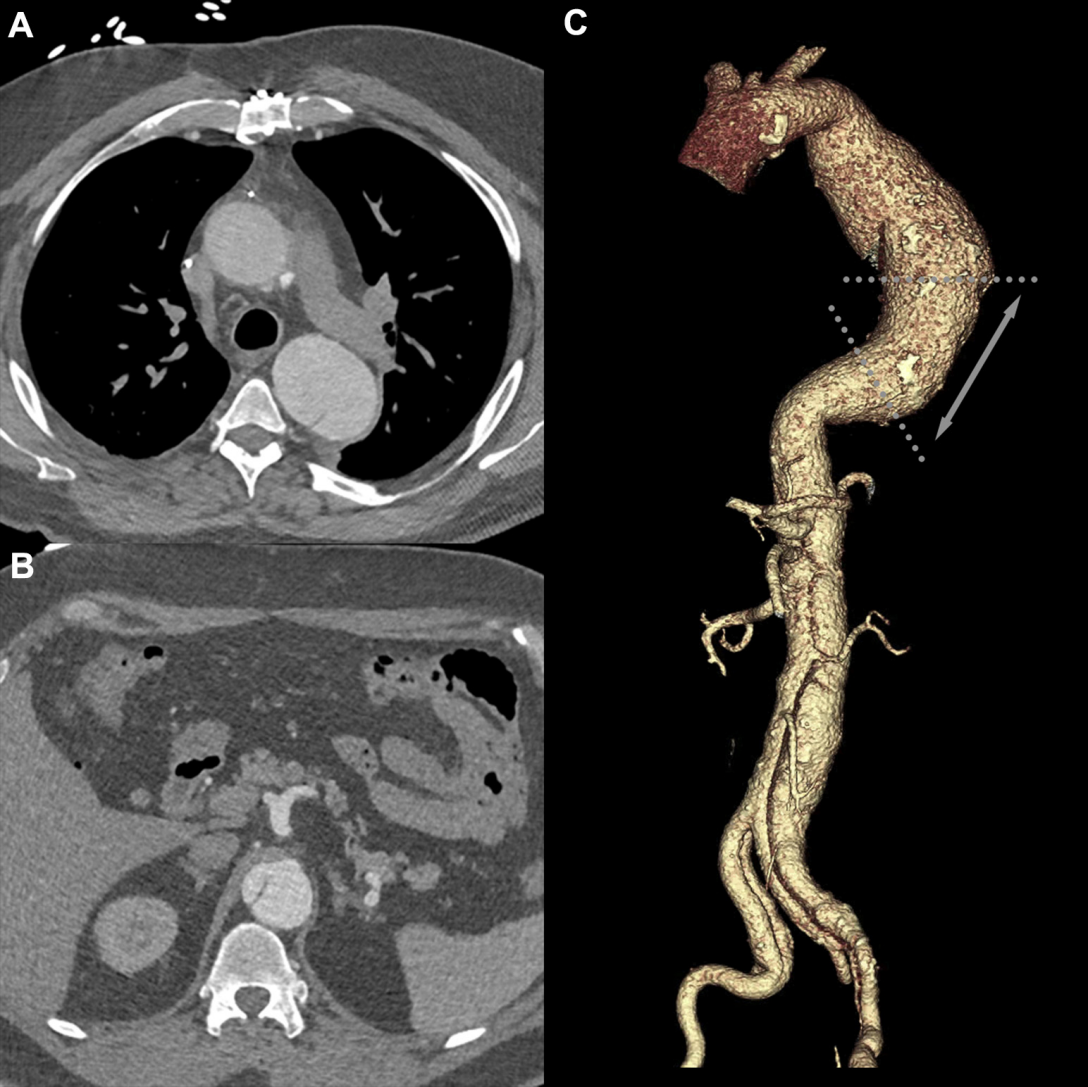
Computed tomography angiogram of a 47-year-old man with aneurysmal degeneration of a chronic type B aortic dissection with a short-segment midthoracic aortic occluded true lumen. A, Proximal extent of dissection with initial entry tear. B, Distal re-entry tear 5 mm cephalad to the celiac artery origin. C, Three-dimensional reconstruction of dissection; *dotted lines* represent extent of true lumen occlusion.
